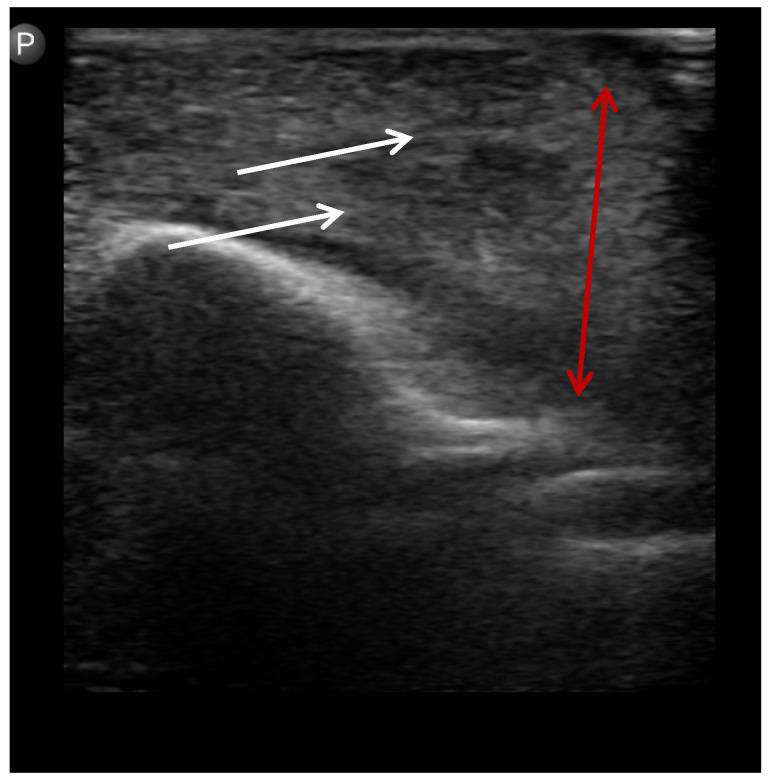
Ultrasound image showing cellulitis. White arrows: diffuse fascial thickening without a discrete collection. Red arrow: infiltration consistent with cellulitis.
